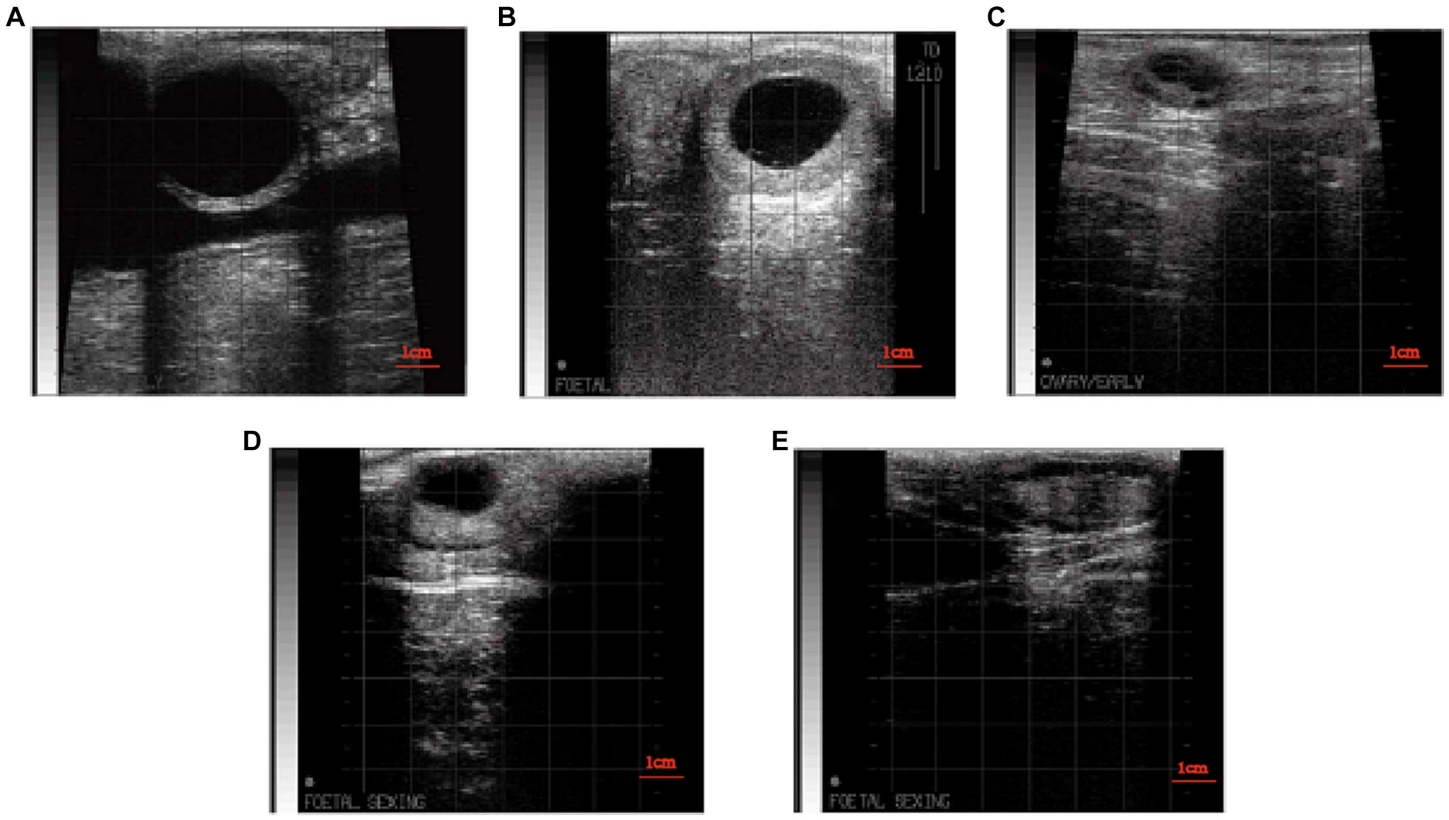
(A) B-mode ultrasound image follicular cysts. (B) B-mode ultrasound image of luteal cysts. (C) B-mode ultrasound image of inactive ovaries. (D) B-mode ultrasound image of dominant follicle after treatment of ovarian disease. (E) B-mode ultrasound image of dominant follicle after ovulation.
